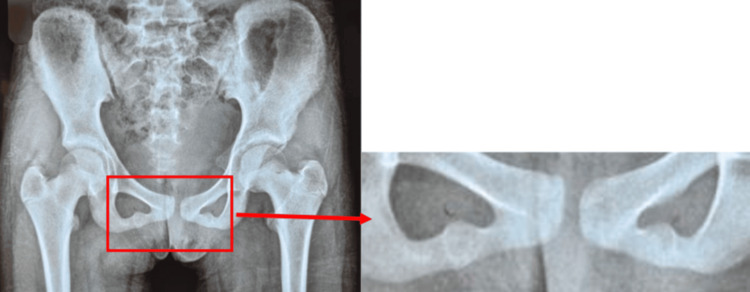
X-ray of the pelvis with both hips anteroposterior view showing bilateral ischiopubic synchondrondritis.
